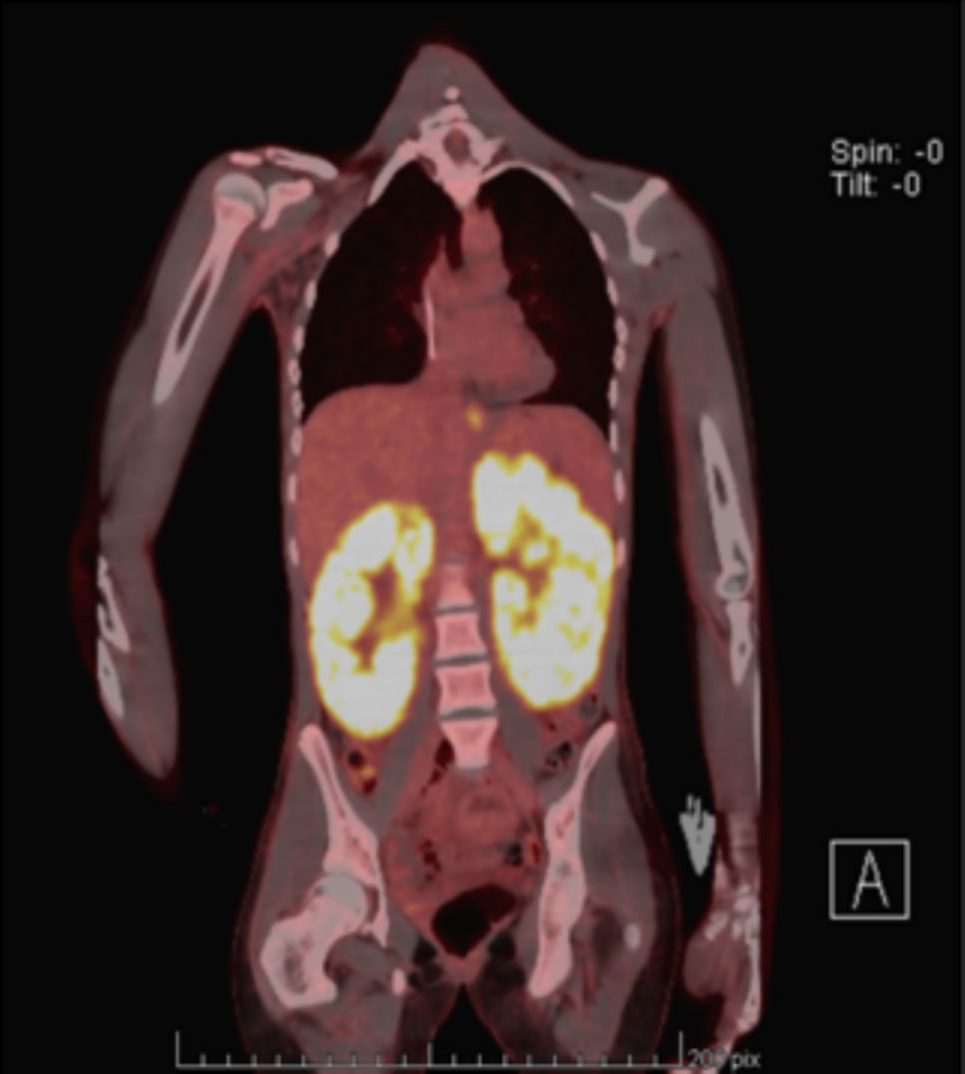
FDG-PET/CT scan showed bilateral enlarged hypermetabolic kidneys without extrarenal hypermetabolic region
